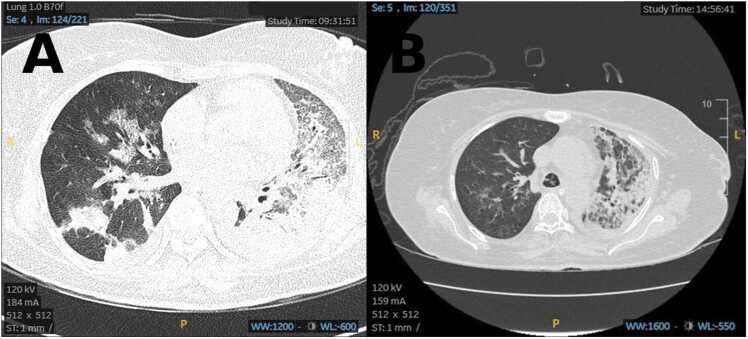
Axial computed tomography images of the thorax obtained during hospitalisation. (A) CT scan on Day 5 of admission demonstrating extensive bilateral parenchymal opacities with predominant left-sided involvement. (B) CT scan on Day 26 showing interval radiological improvement with partial resolution of parenchymal changes.
